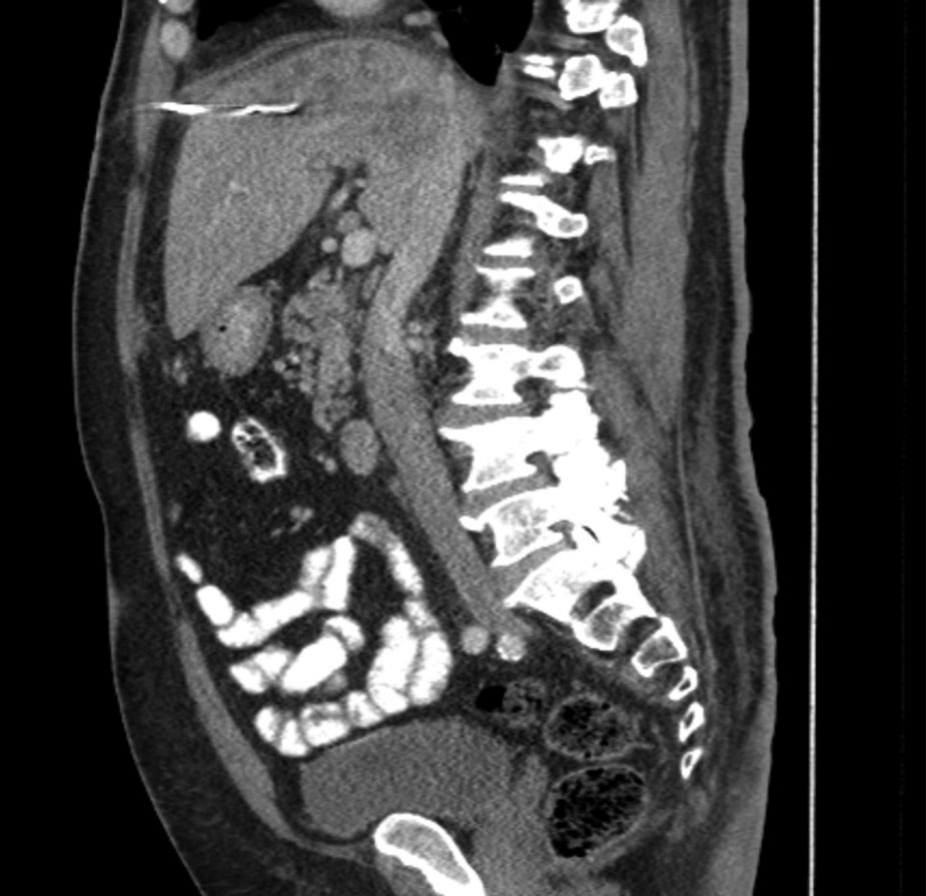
Abdominal and pelvis computed tomography with oral and intravenous contrast depicting complex loculated collection within the liver and a pigtail catheter placed (sagittal view)
